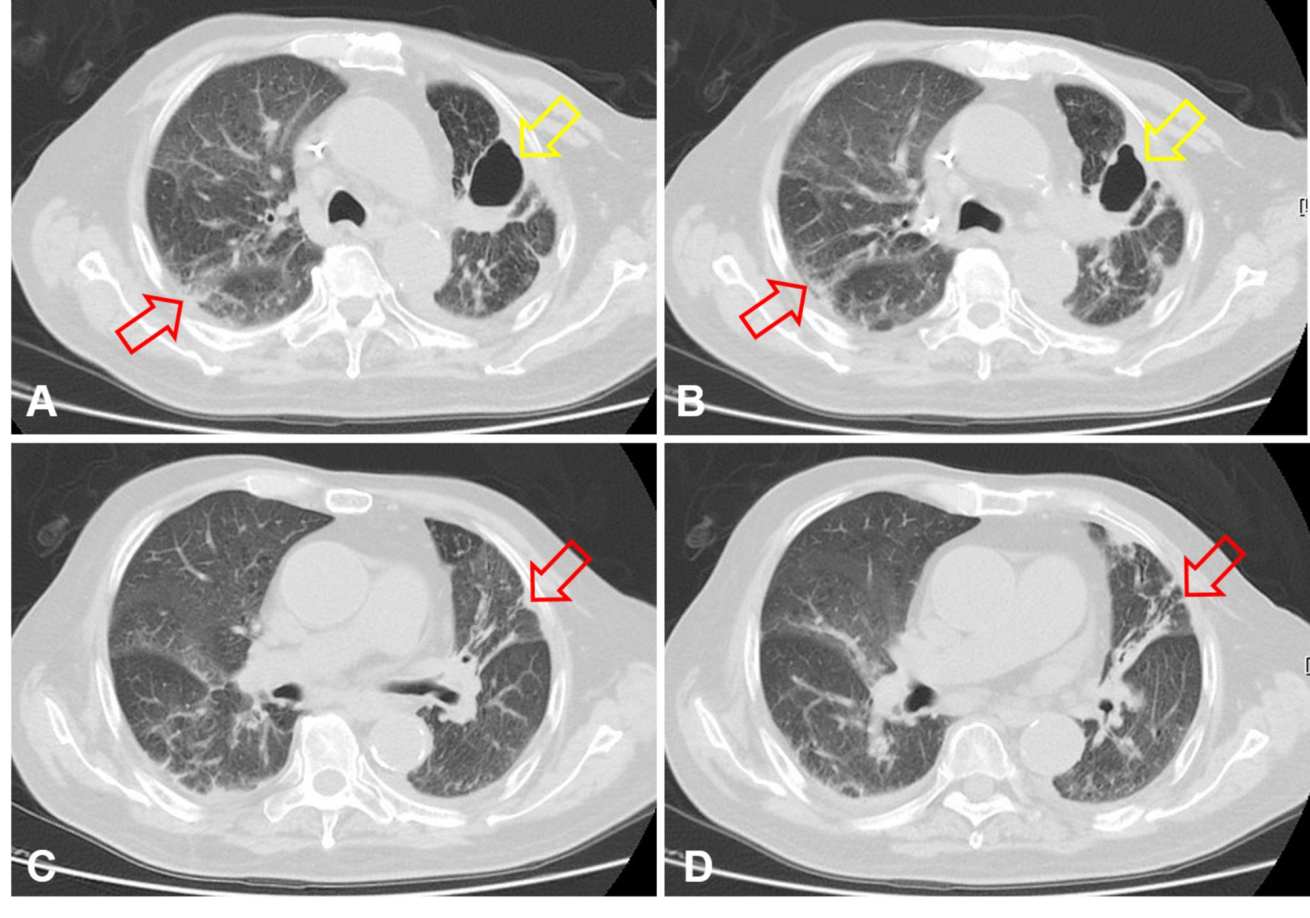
Progressively shrunken tumor cavity (yellow arrows) and much improvement of the pneumonitis with residual fibrotic changes (red arrows) on sequential axial CT scan (lung window) as compared with Figure 7: A to D, September 9, 2019
